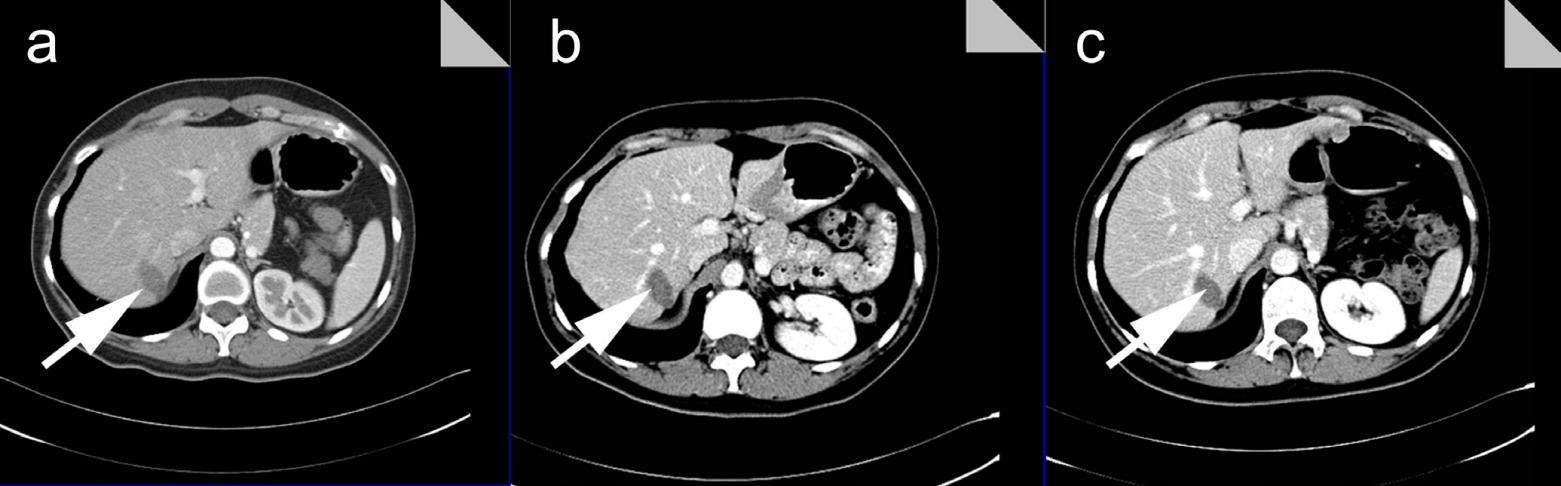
Post-operative CT imaging in a cohort of MWA patients a. After one month post-treatment with MWA, the CT imaging showed the low intensity of lesions without peripheral enhancement; b. After three months post-treatment with MWA, the CT imaging showed the low intensity of lesions without peripheral enhancement; c. After six months post-treatment with MWA, the CT imaging showed much lower intensity of lesions without peripheral enhancement than ever.
